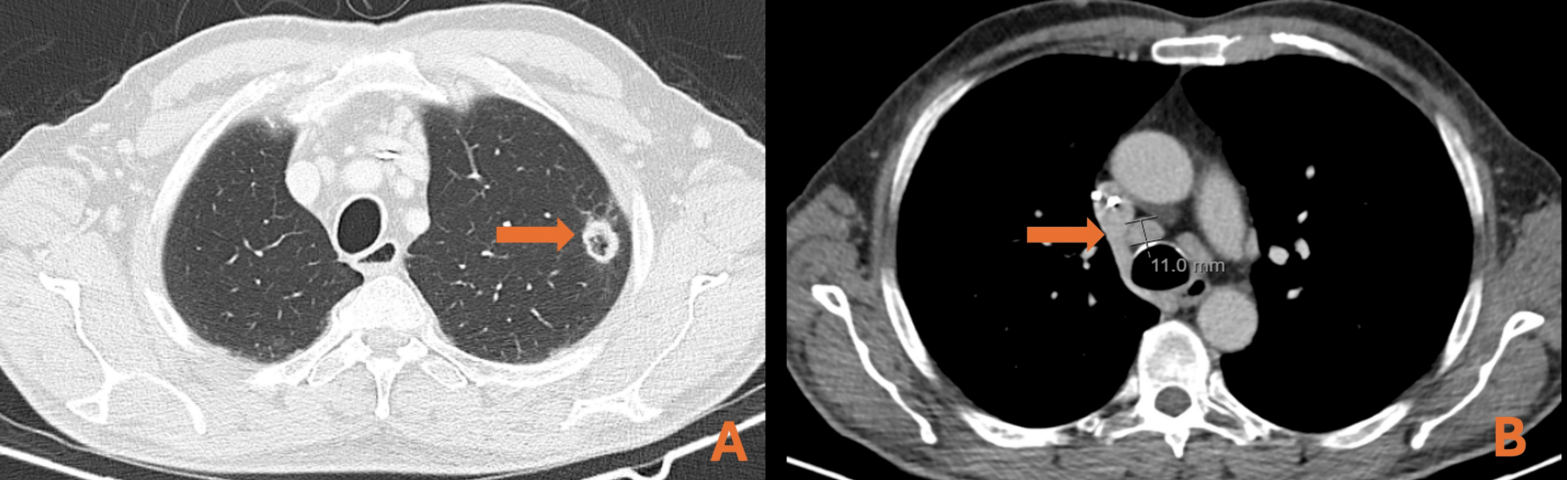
CT of the chest, abdomen, and pelvic showing (A) the pulmonary lesion and (B) the de novo nodes CT: computed tomography
